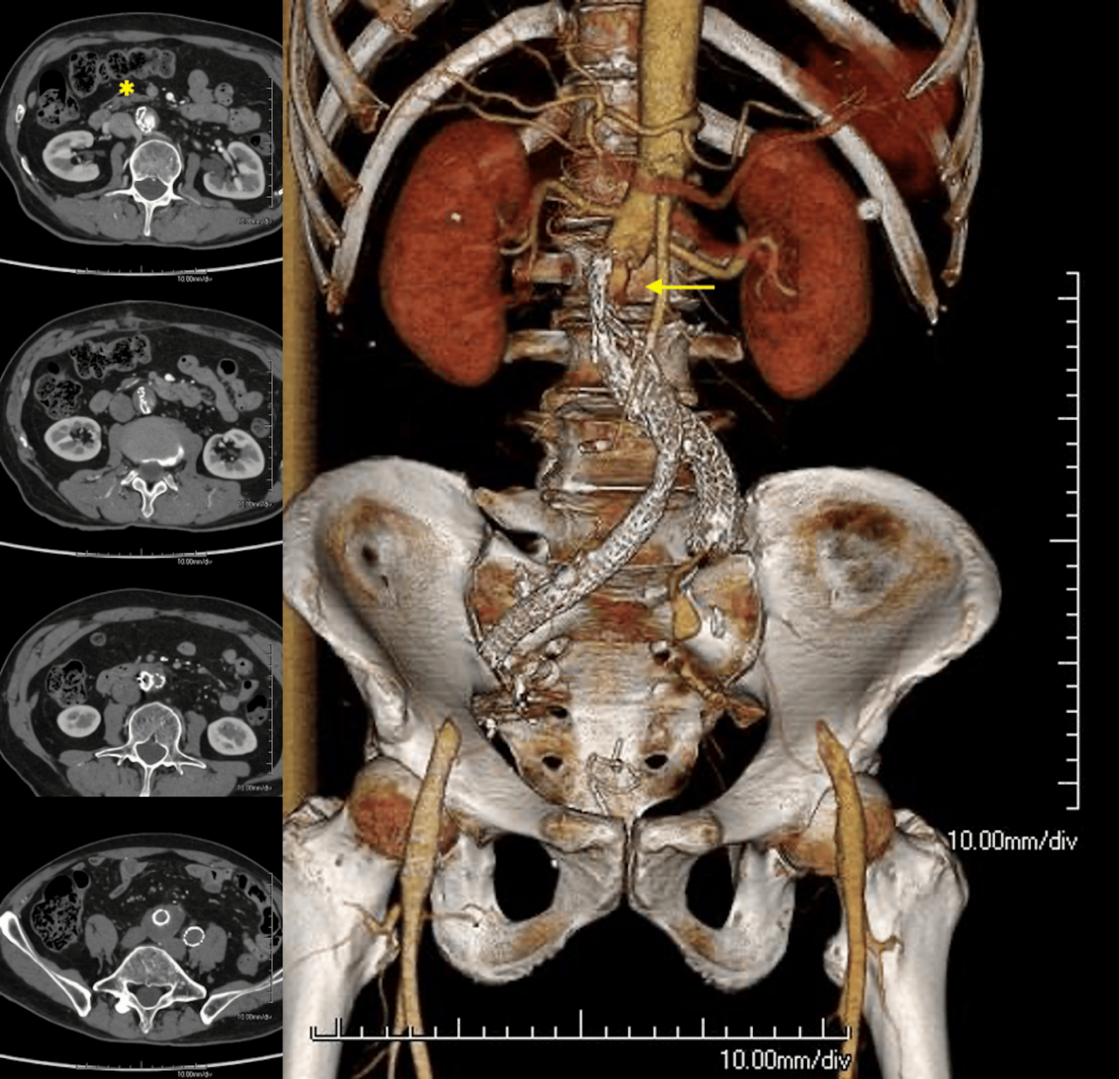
Axial sections and volume-rendered reconstruction of CTA one month after EVAR The arrow indicates the collapse of the proximal endograft, which caused acute aortic occlusion and thrombosis of the bilateral common iliac limbs. The axial image demonstrates the collapsed main body (^*^). CTA, CT angiography; EVAR, endovascular aortic aneurysm repair
